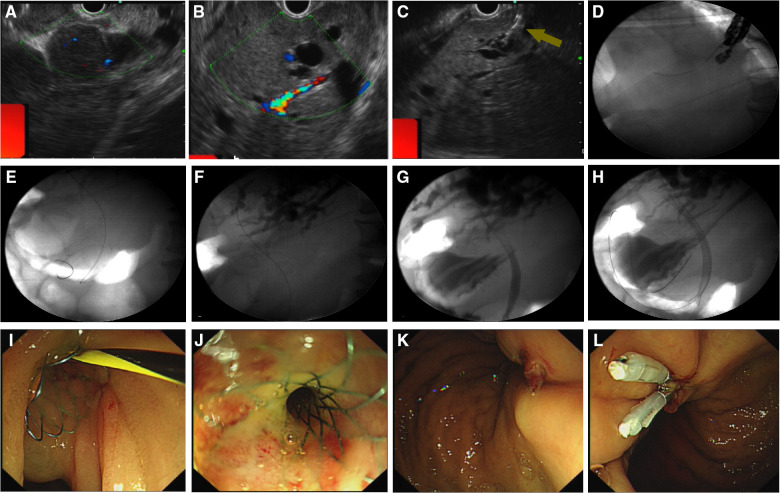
Endoscopic ultrasonography–guided biliary drainage. (A) Linear-array ultrasonography showed numerous filling lymphomatous nodules in the abdominal cavity. (B,C) The left hepatic duct was located for puncture. (D,E) The guidewire was passed through the confluence of the left and right hepatic ducts, and then through the site of common bile duct stricture. (F,G) Radiography indicated that the stricture site at the upper common bile duct was 4 cm away from the hepatic portal. (H) A bare metal stent (8 cm × 10 mm) was placed transgastrically in the common bile duct in an antegrade manner. (I,J) Gastroscopy demonstrated that the stent was passed through and stretched exactly out of duodenal papilla. (K,L) The puncture point in the stomach was closed with a titanium clip.
